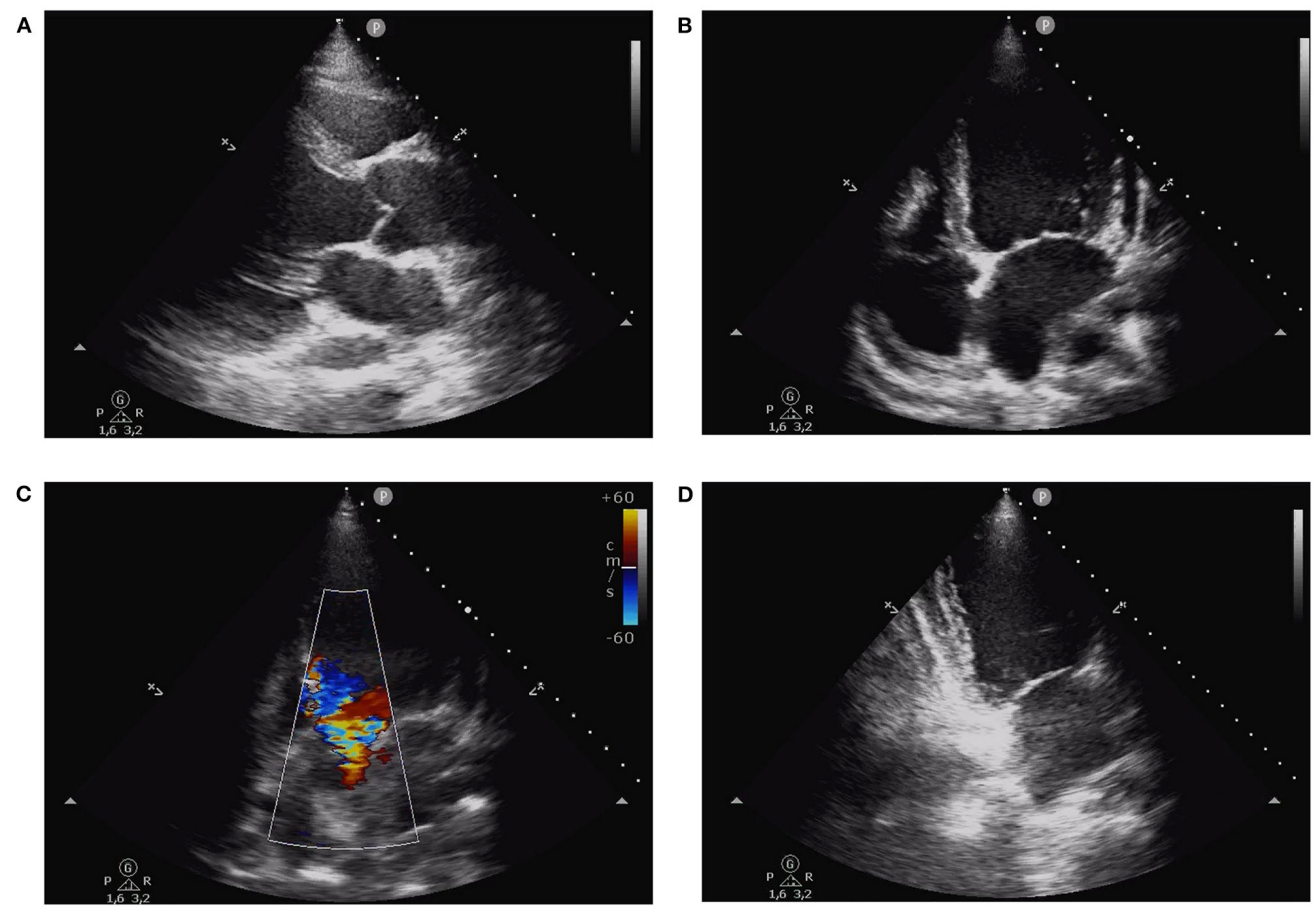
Transthoracic echocardiogram obtained at admission. (A) Parasternal long axis view showing left ventricle dilation and dilation of aortic root and ascending aorta. (B) Apical four-chamber view revealing left ventricle dilation with reduced ejection fraction due to apical and midventricular akinesia in the septal and lateral walls, and normal right ventricular function. (C) Severe aortic regurgitation in (five-chamber view). (D) Apical two-chamber view showing akinesia of the midventricular and apical segments of the anterior and inferior left ventricular walls.
